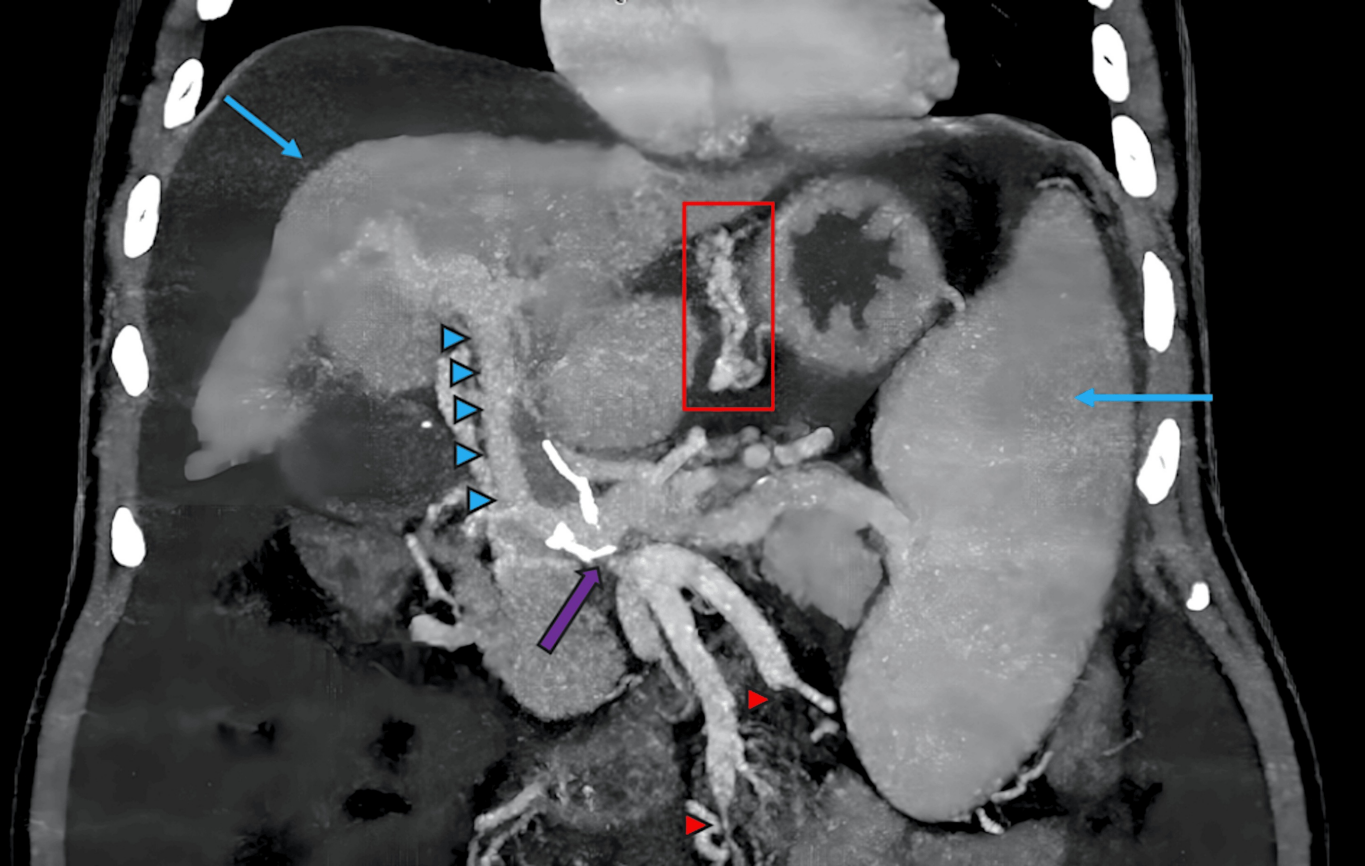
Pre-procedure contrast-enhanced computed tomography image The image shows the classical signs of chronic liver disease—nodular liver with right lobe atrophy and splenomegaly (blue arrows). Extra-mural gastric varices are marked with a red rectangle. Portal hypertension is marked with blue arrowheads; signs of portal hypertension are readily apparent. The SMV-IMV conjoint trunk ostio-proximal stenosis is indicated by a purple arrow. Both SMV and IMV show chronic thrombus and short-segment CTO (red arrowheads). These were later confirmed with diagnostic digital subtraction angiography. SMV: superior mesenteric vein; IMV: inferior mesenteric vein; CTO: chronic total occlusion
